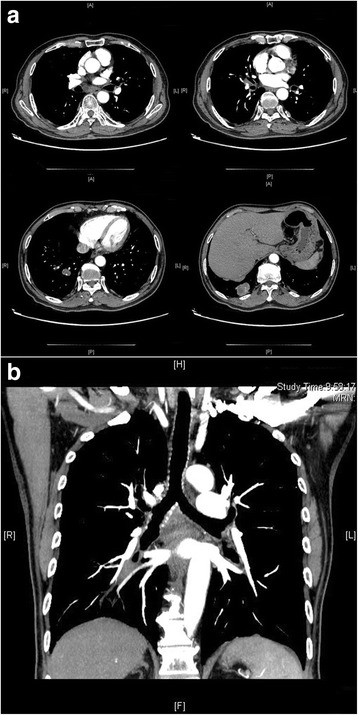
a Series of CT images showed two lesions in *right lower* lobe with enlarged subcarinal, *right* hilar and interlobar lymph nodes. b Coronal CT image showed the relationship between the subcarinal lymph nodes and the airways
